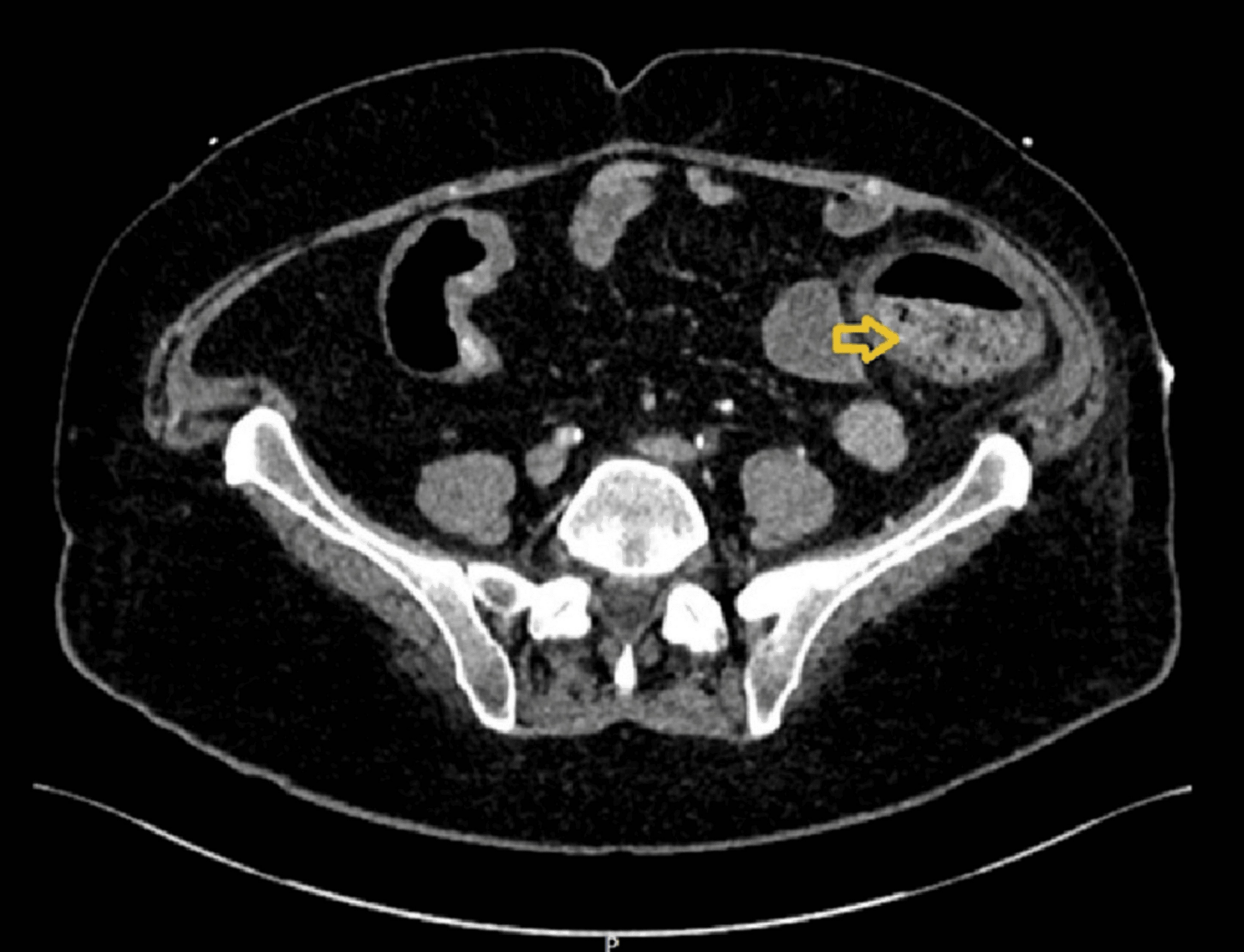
Vascular watershed ischemia in the descending colon The above CT scan (yellow arrow) revealed that the descending colon in a vascular watershed zone showed thickening and peri-colonic fat stranding in keeping with acute ischemia.
